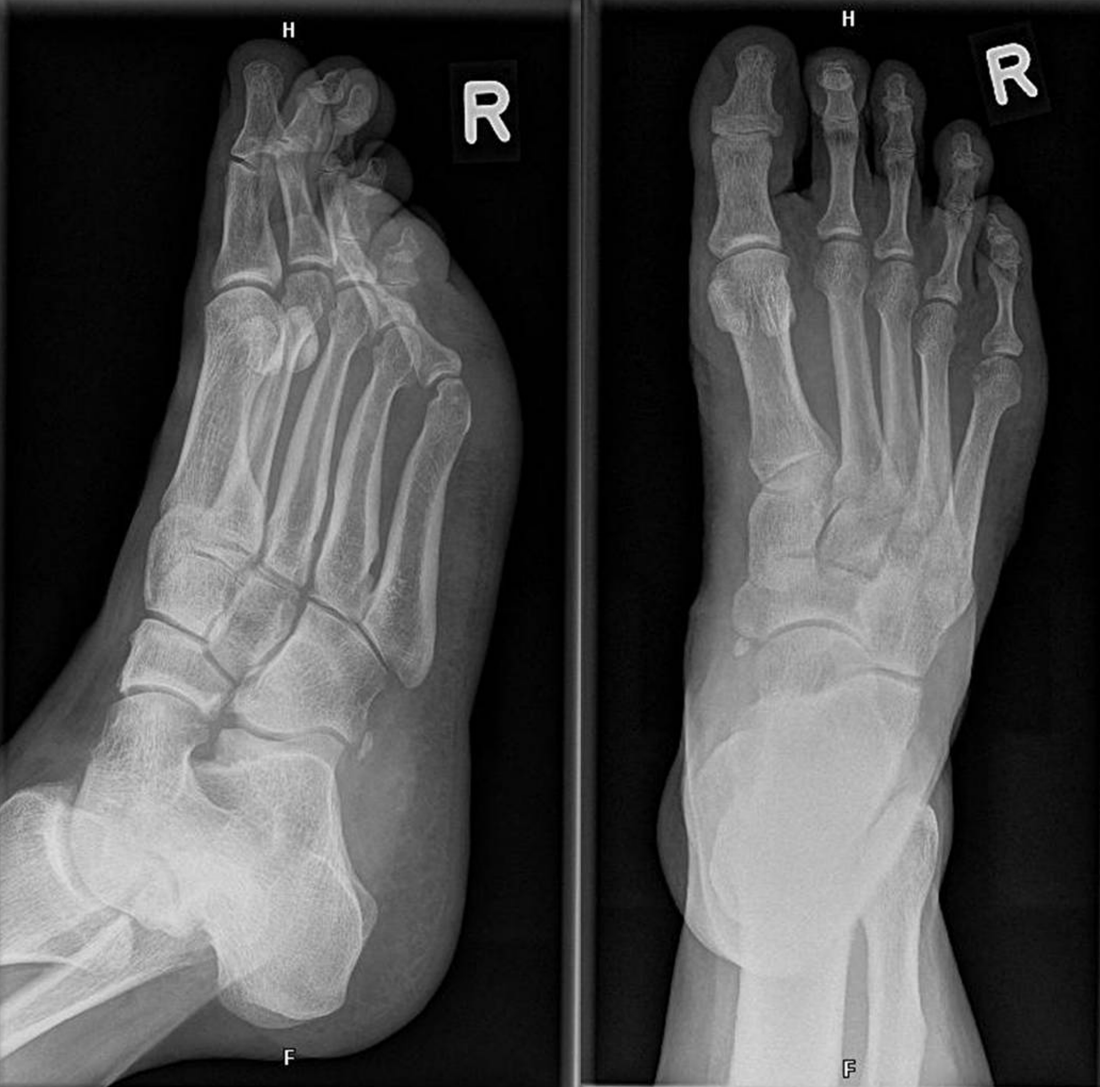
Scan of the calcaneus with a discrete fracture without dislocation of the bone: Conventional X-ray taken at the initial examination
